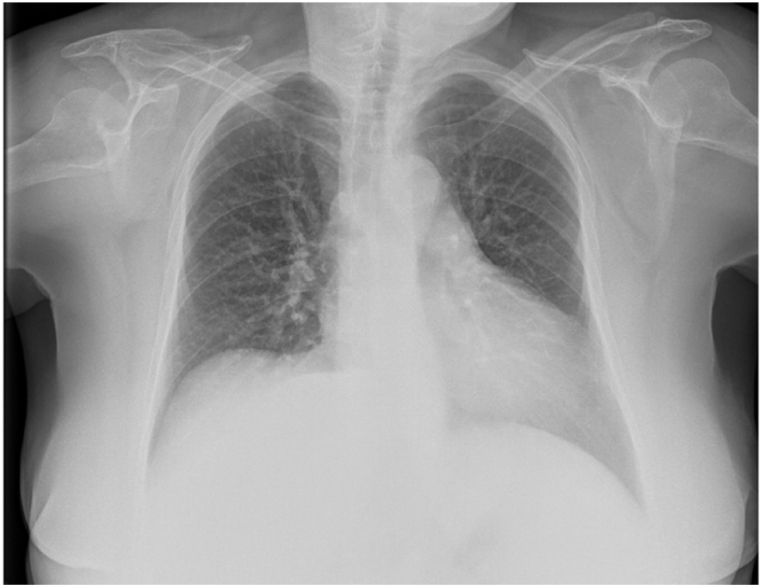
Evidence of peribronchial cuffing and hilar congestion at the Chest X-Ray.
